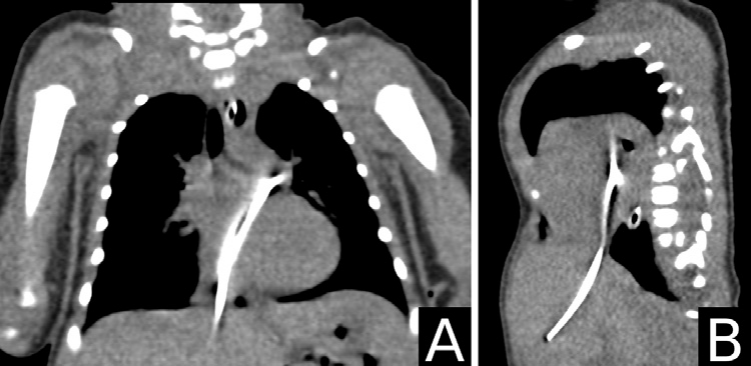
Coronal (A) and sagittal (B) reconstructed computed tomography (CT) images show a clear view of an opaque cylindrical foreign body representing the retained catheter fragment.
